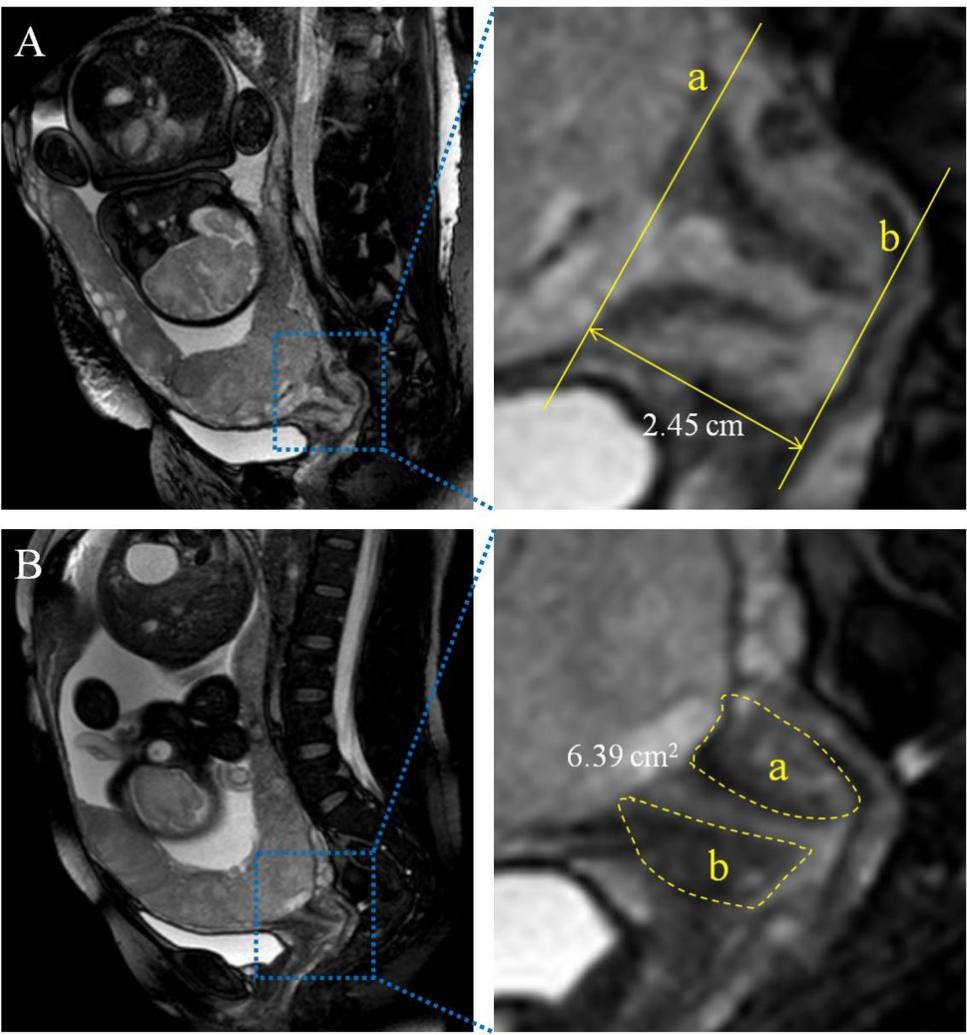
A Cervical length was measured between lines a and b in sagittal magnetic resonance imaging (MRI) image (2.45 cm). B Cervical area was measured by Image J software in sagittal MRI image (yellow circles a and b) (6.39 cm^2^)
